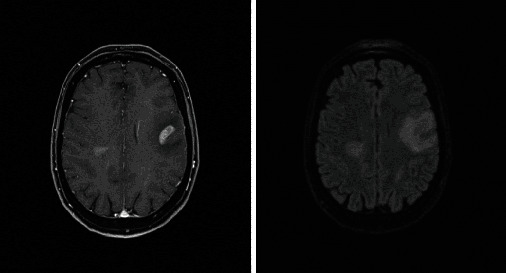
Irregular mass involving the left frontal lobe. Radiographic differential diagnosis: metastasis versus primary CNS neoplasm.
